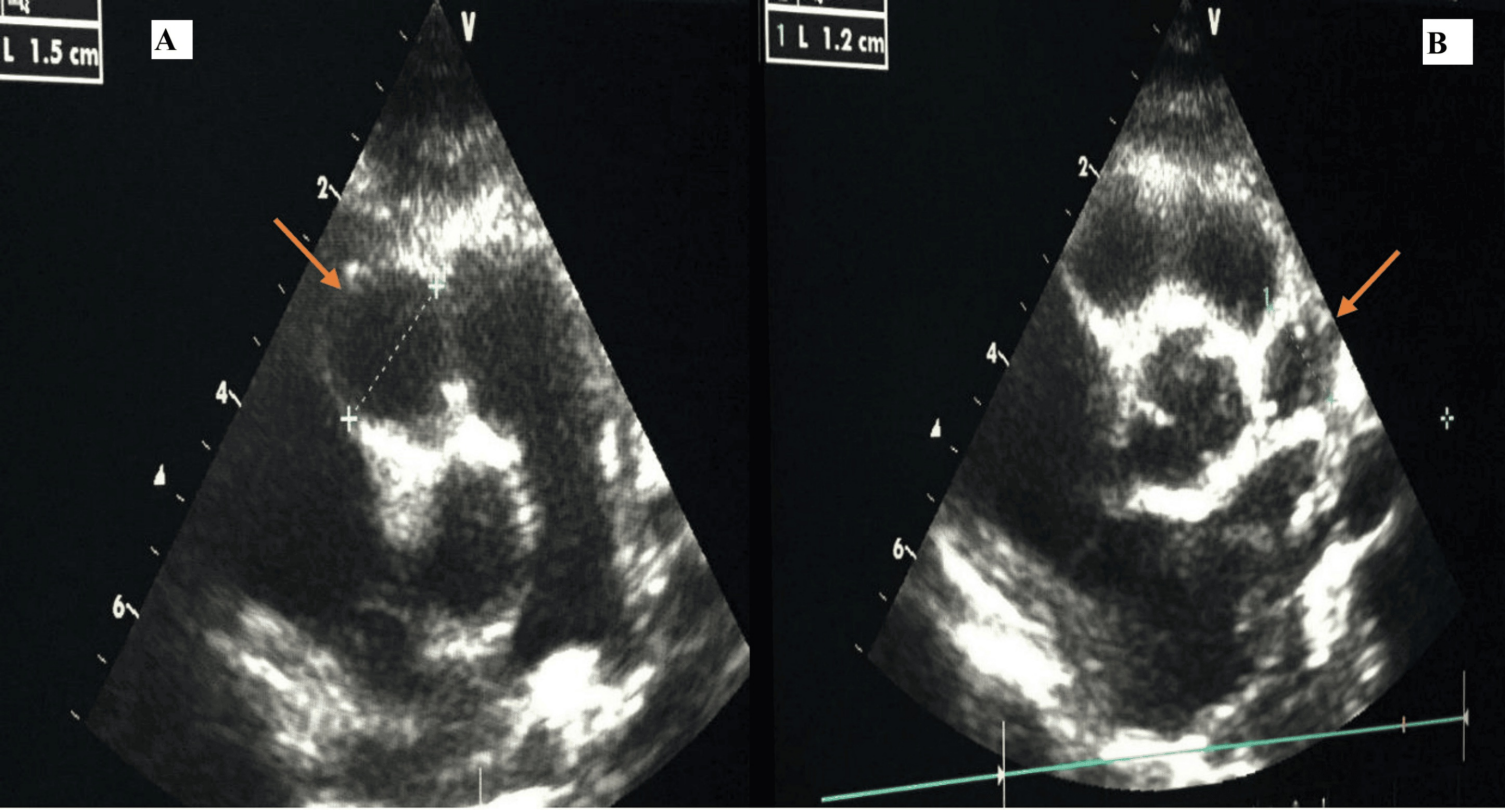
Echocardiogram Revealing Fusiform Aneurysms in Coronary Arteries: 15 mm in the Right and 12 mm in the Left
